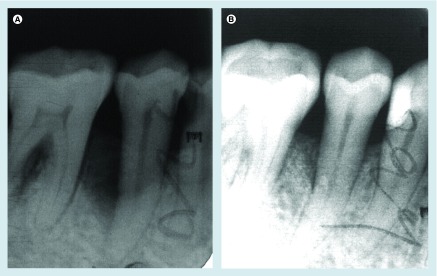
Periapical radiograph of the second right mandibular premolar 1 year post-surgery.
